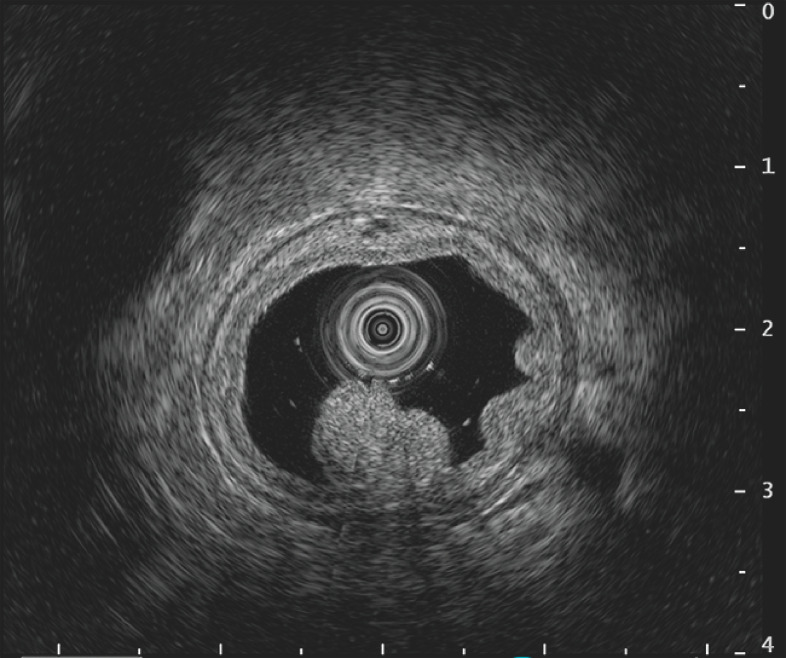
Endoscopic ultrasound image showing that the duodenal muscularis propria was preserved in a circular shape and was not adhering to the involutions of the folds.
